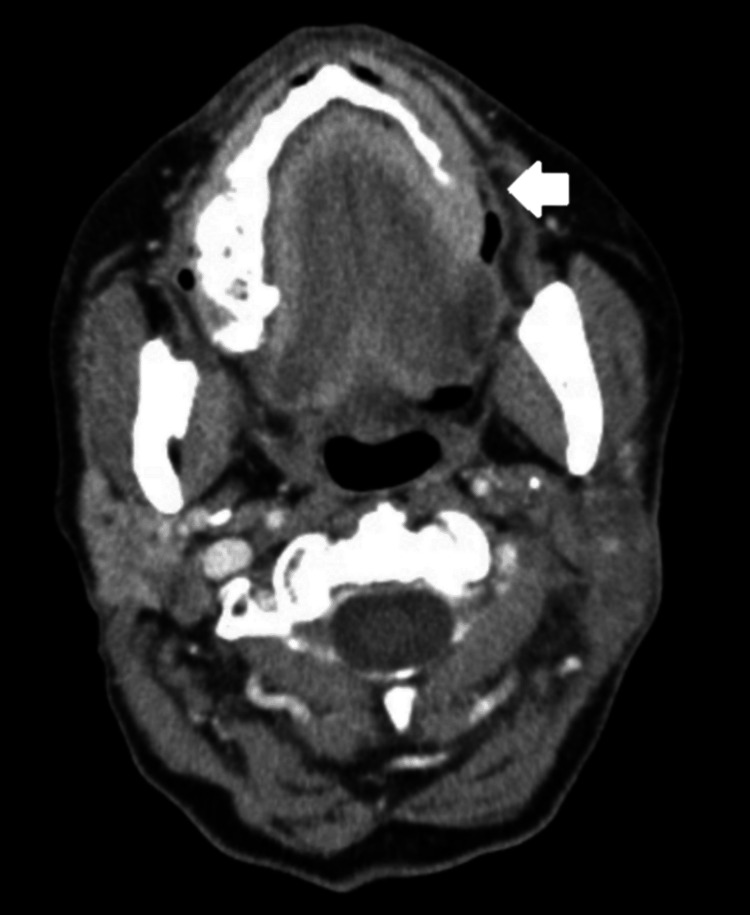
CT neck soft tissue with IV contrast Long-standing postoperative changes from a left neck dissection (white arrow)
